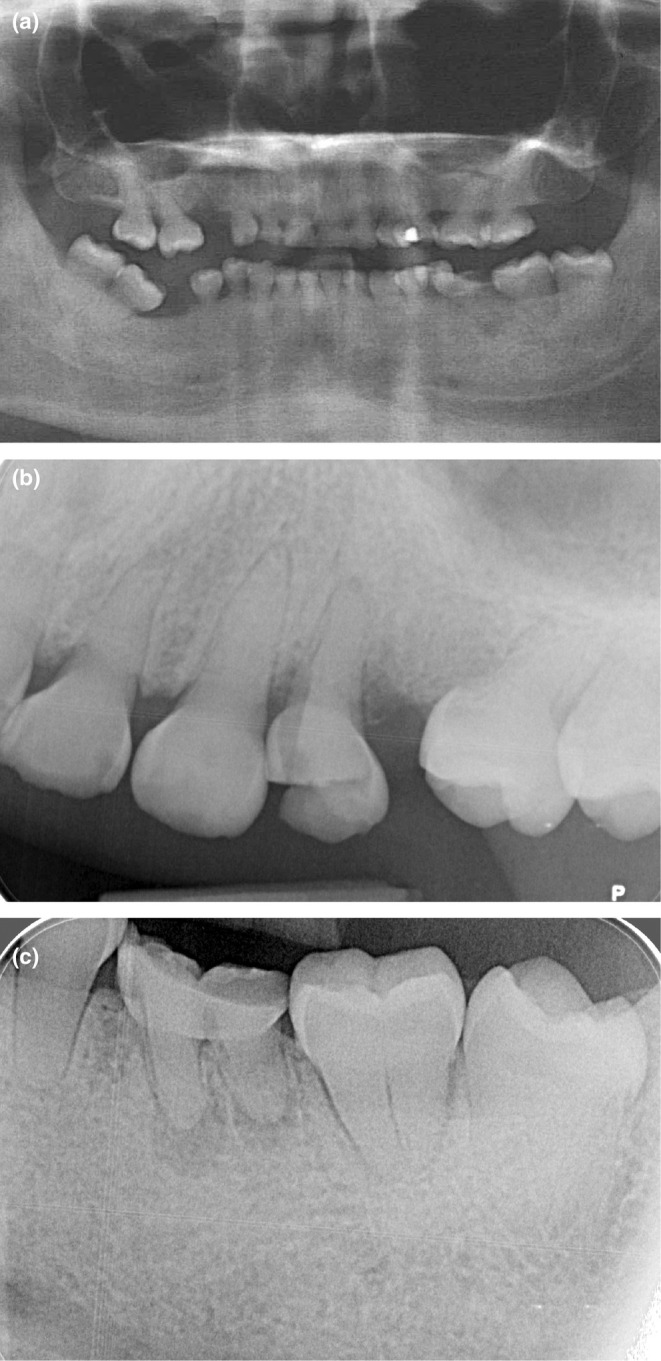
Initial radiographs (a) Initial orthopantomogram, all teeth display distinctive characteristics of DI, with globular crowns, cervical constrictions, and short, thin roots. Pulpal obliteration is observed. (b) Periapical radiograph of sector 2, a radiolucent image is present around the apex of tooth 25. (c) Periapical radiograph of sector 3, tooth 36 exhibits a periapical lesion involving the apices of both roots. Teeth 24 and 36 are to be extracted.
